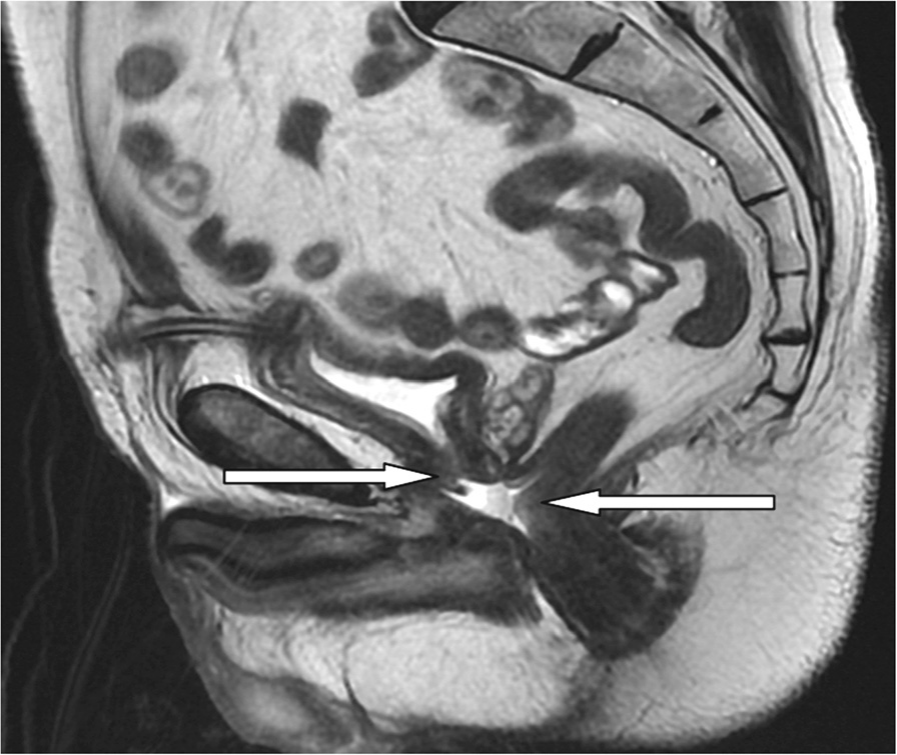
Magnetic resonance image demonstrating moderate sized rectourethral fistula (arrows).
